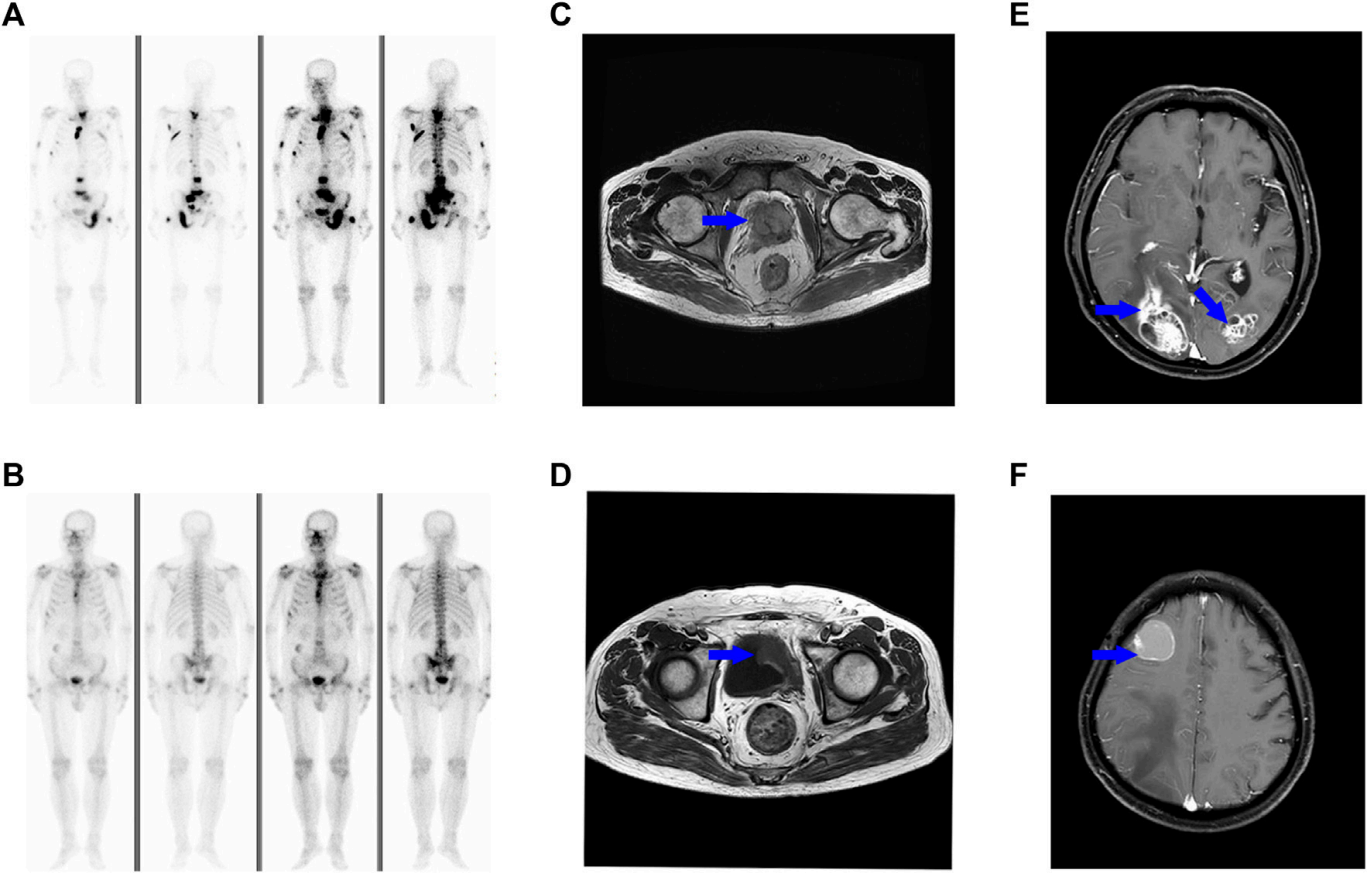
Bone scan shows the patient developed multiple loci of metastasis in the lumbar vertebra, sacral vertebra and left pubis (A). The metastatic lesions response well to ADT (B). MR images show a soft tissue mass in the pelvis [(C), blue arrow], and the lesions partially regress after three cycles of chemotherapy [(D), blue arrow]. A brain MRI scan reveals multiple metastases [(E, F), blue arrow].
